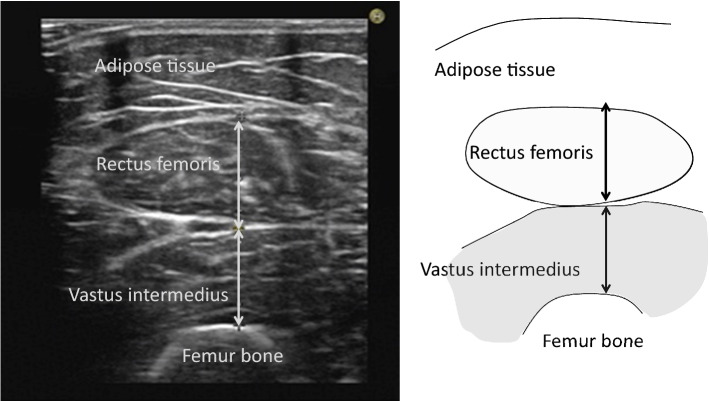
Ultrasound image of the rectus femoris and vastus intermedius.
